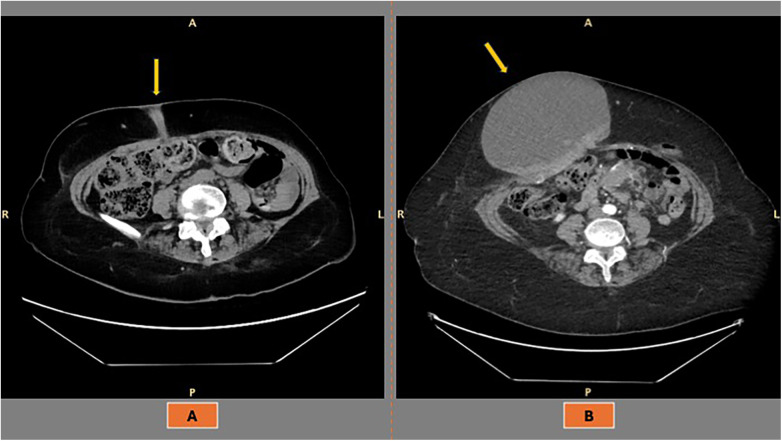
A: recurrence of ovarian cancer at the port site, presenting at CT scan as a small lesion in the subcutaneous tissue of the anterior abdominal wall, extending from the skin to the underlying muscle. B: The previously described lesion progressed into a giant metastatic mass.
